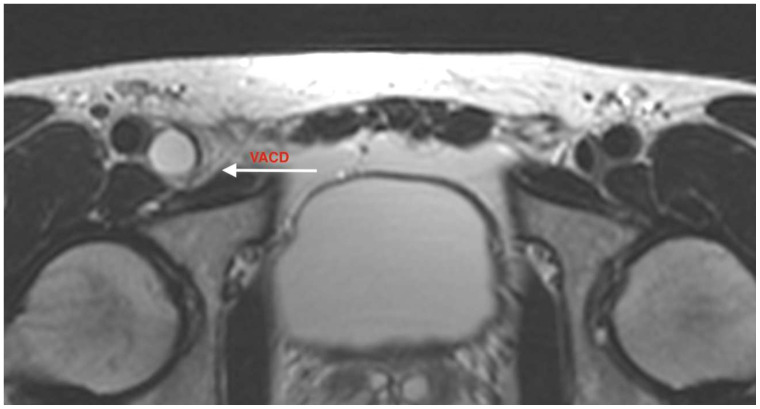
Cross-sectional magnetic resonance imaging demonstrating venous adventitial cystic disease with homogeneous low signal intensity on T1-weighted images and high signal intensity on T2-weighted images, consistent with mucinous content. The irregular homogeneous structure fills almost the entire lumen. The arrow indicates the cystic irregular lesion.
